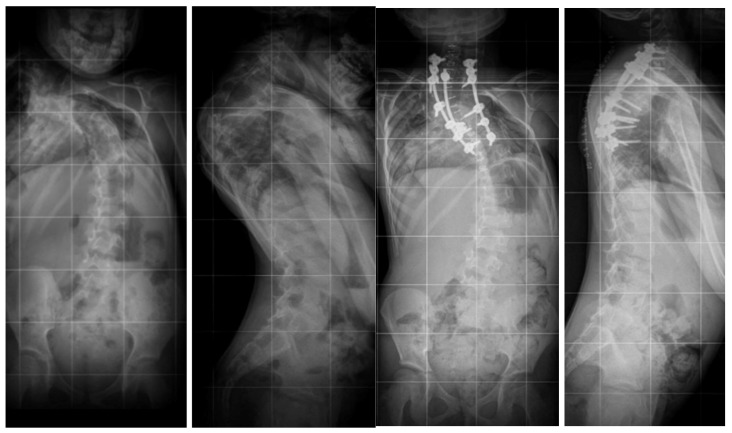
X-rays of 5-year-old girl with congenital progressive and rigid kyphoscoliosis treated with posterior vertebral column resection at T3–T4 level.
